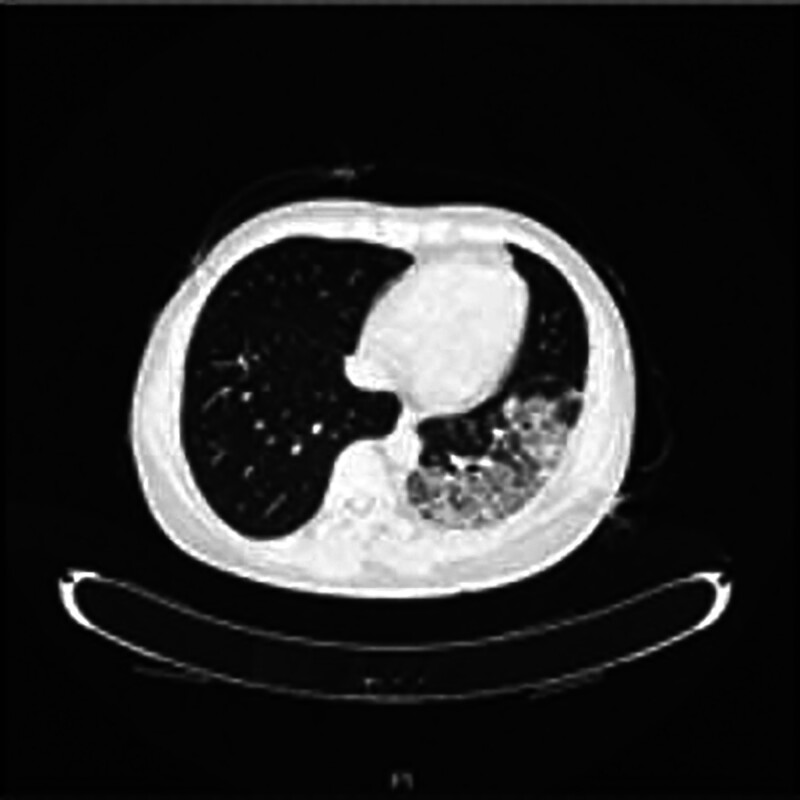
Patient was reexamined using pulmonary CT on November 14, 2023. CT = computed tomography.
